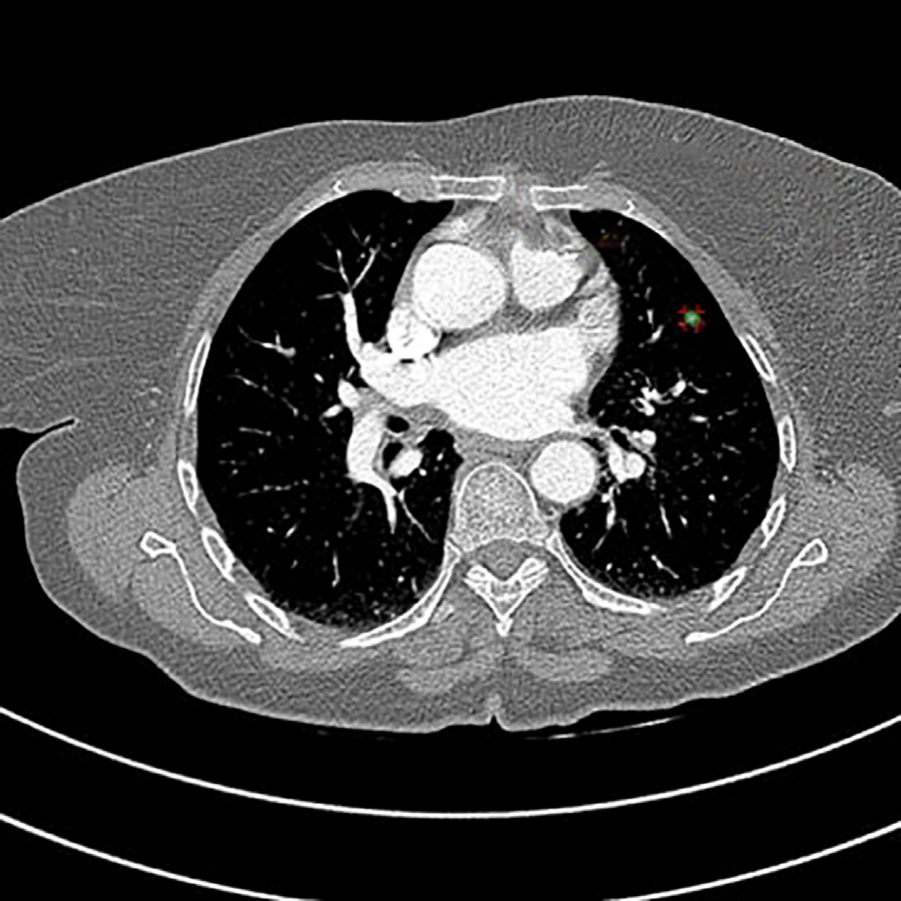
Axial HRCT chest showing well-defined lobulated left lower lobe pulmonary nodule, measuring 137 HU in density, with no calcification, suspicious of metastasis.
